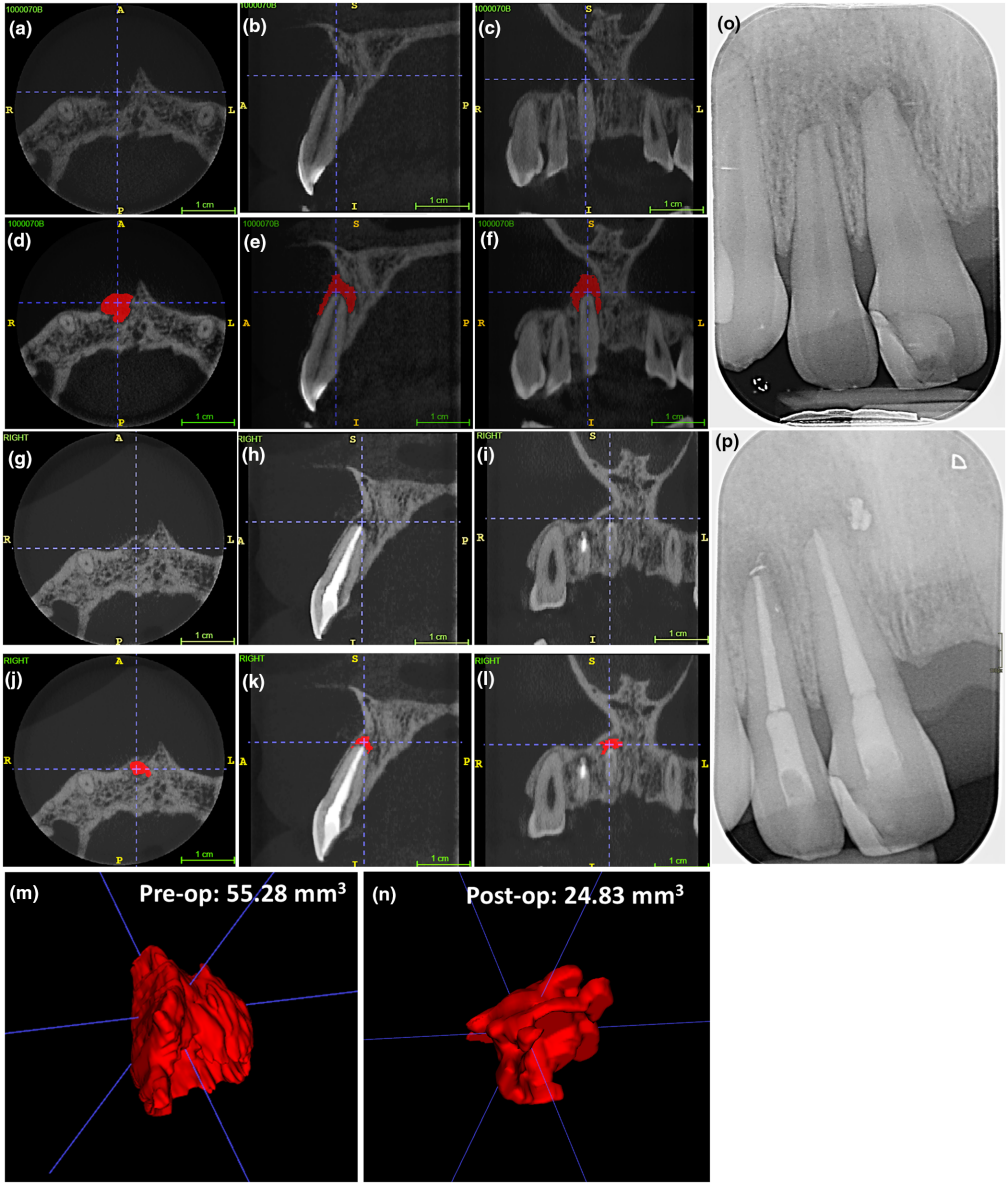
Longitudinal volumetric cone beam computed tomography analysis of periapical lesions following root canal treatment of the UR1. The example represents the axial [a,d,g,j], sagittal [b,e,h,k] and coronal [c,f,i,l] views alongside the pre‐ [m] and postoperative [n] volumetric analysis of a lesion that has reduced in size following 17% EDTA irrigation at the 12 months review interval [g–l]. The corresponding pre‐ and post‐operative periapical radiographs are depicted in [o] and [p], respectively. Scale bars represent 1 cm.
